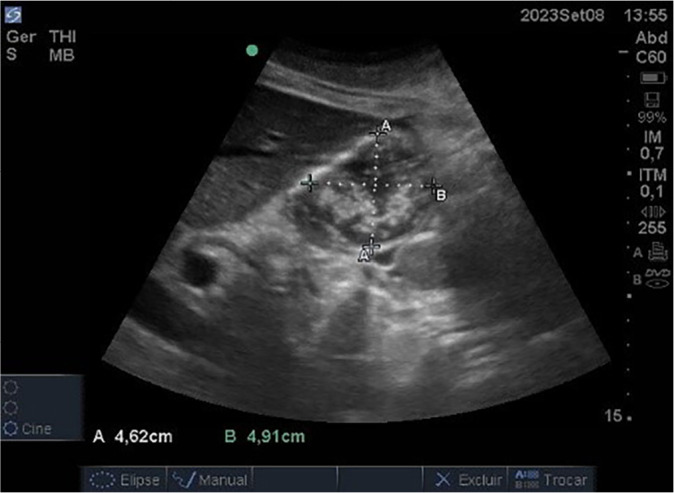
Gastric antrum ultrasound image on intensive care unit day 25
